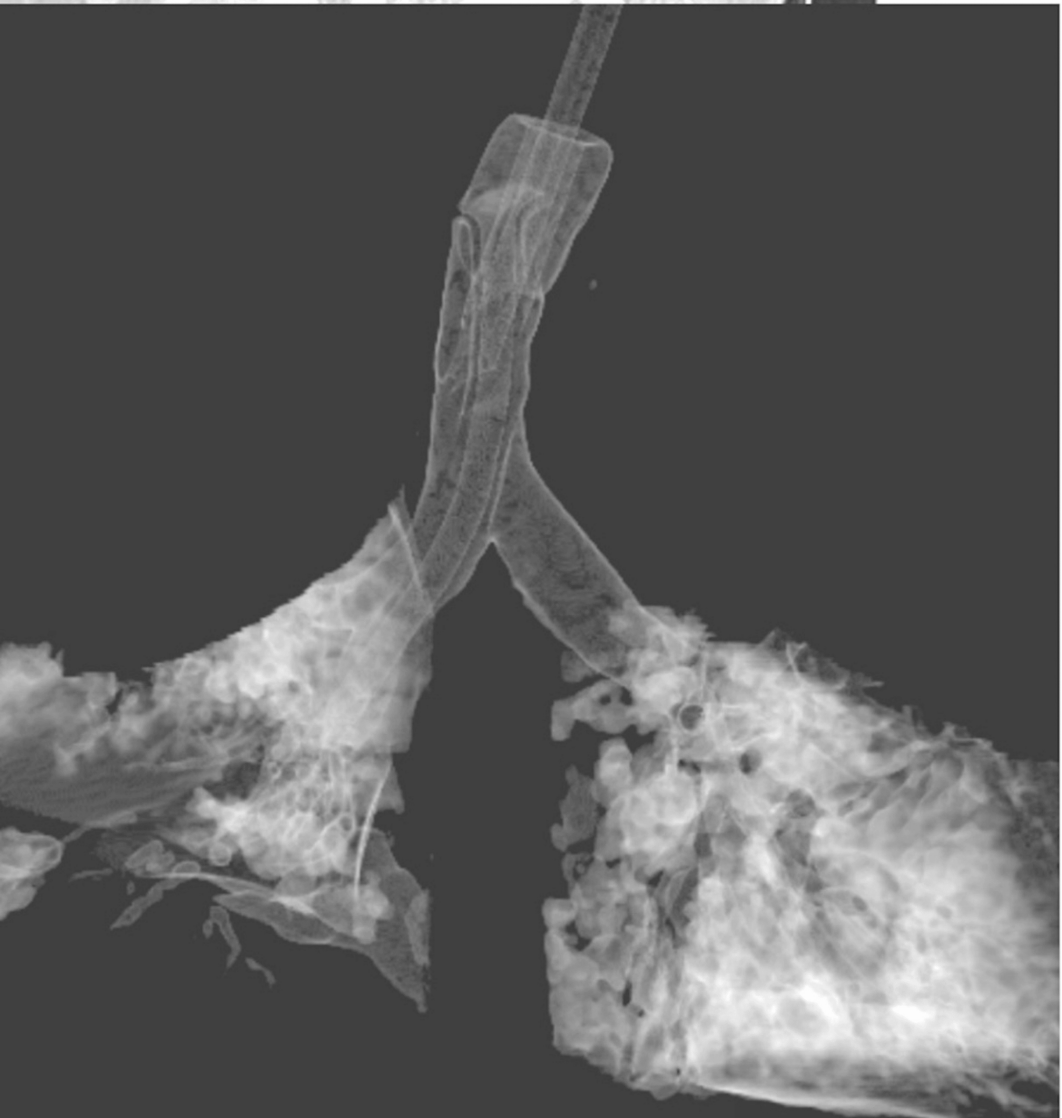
Chest CT reconstructed to show the nasopharyngeal airway.
